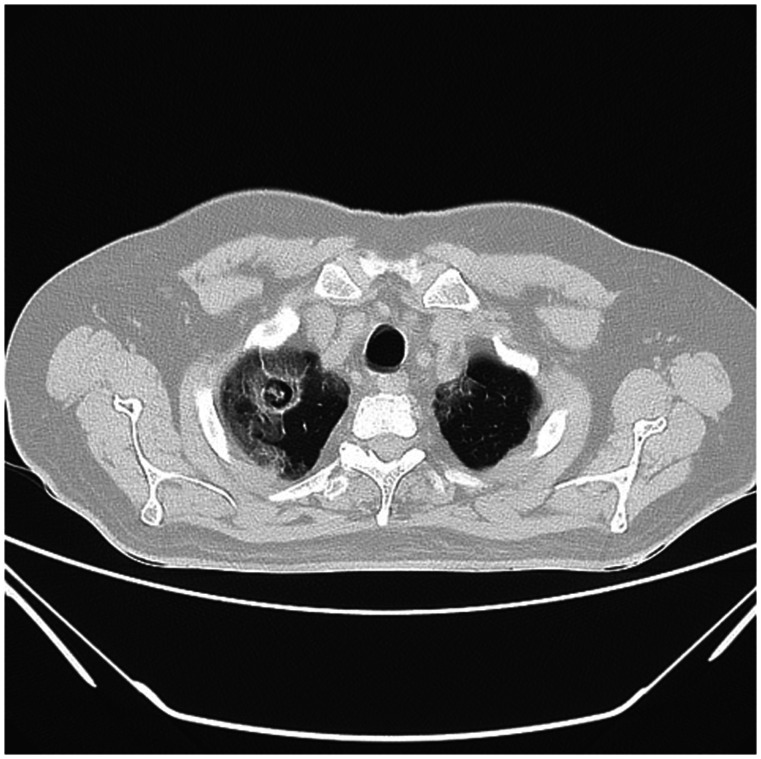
High-resolution computed tomography chest showed bilateral peripheral ground-glass opacities with a right upper lobe cavity measuring 2.3 × 2.3 cm containing an opacity inside which was likely a fungal ball.
